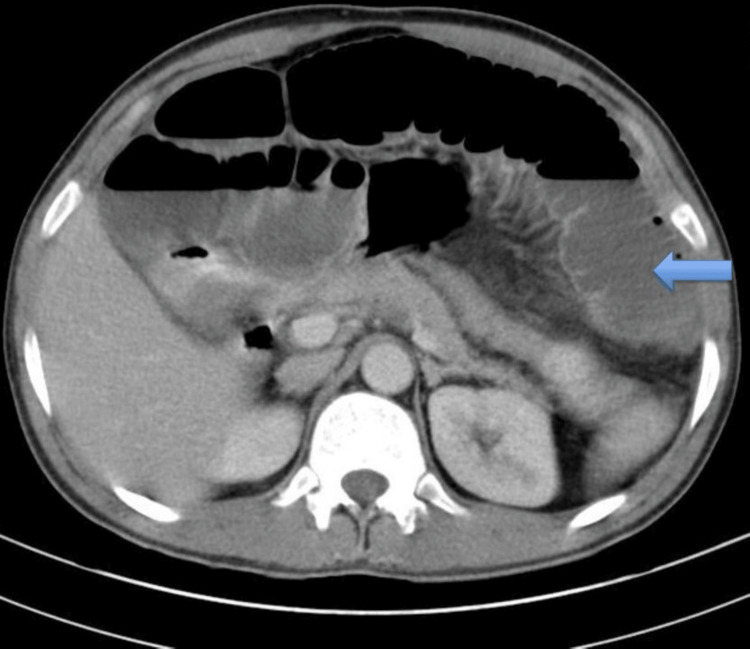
CECT axial view of the abdomen with the blue arrow, demonstrating small intestinal obstruction CECT: contrast-enhanced computed tomography
